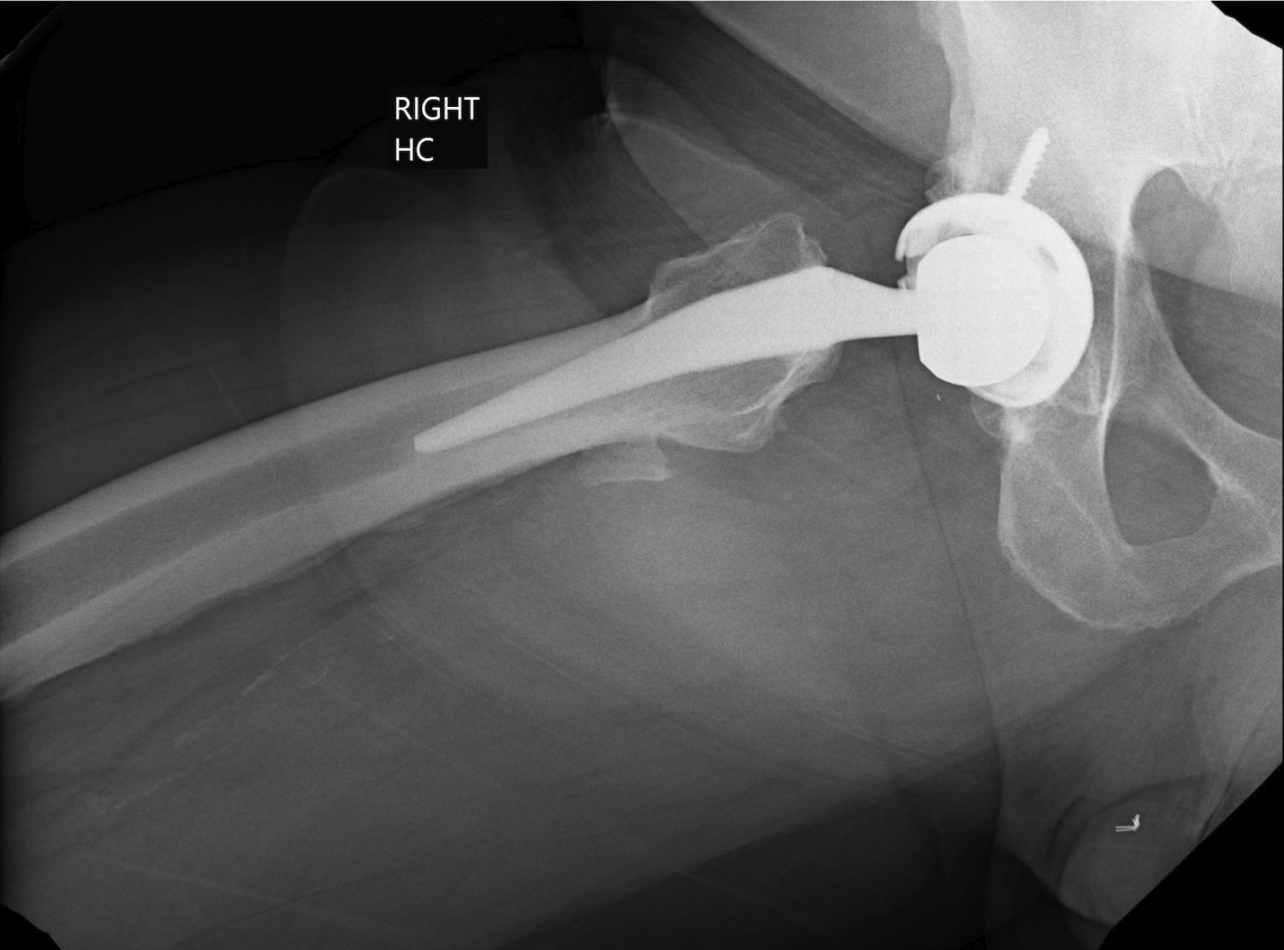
Lateral radiograph of right total hip arthroplasty.
